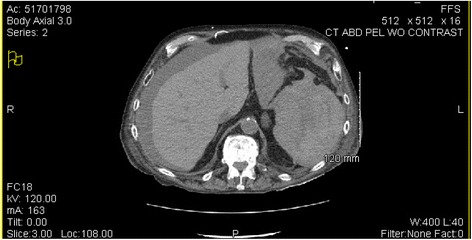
Computed tomography of the patient’s abdomen with splenic rupture and evidence of intra-abdominal bleeding
